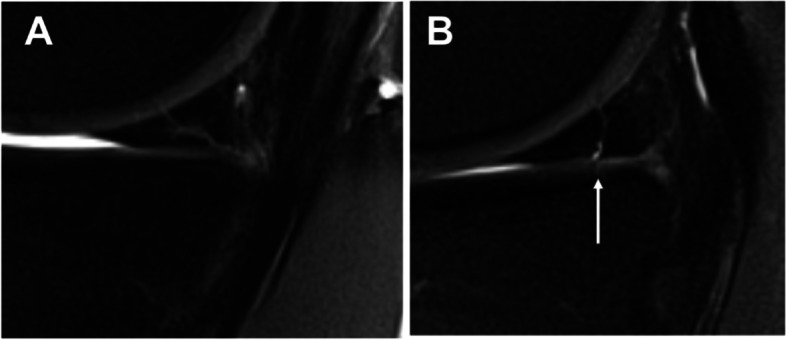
Sagittal fat-suppressed T2-weighted MR images of the knee show (A) a healed medial meniscus with no intrameniscal fluid (Henning type I) and B) a partially healed meniscus with fluid-equivalent signal in less than 50% of the meniscal height (corresponding to Henning type 2)
